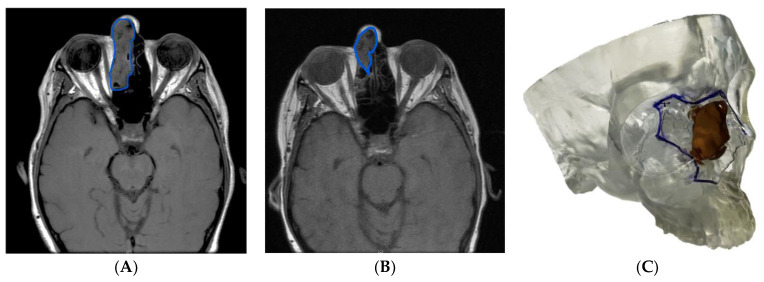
(A) T1-weighted axial MRI pre-induction chemotherapy (blue outline highlighting tumor), (B) T1-weighted axial MRI post-induction chemotherapy, and (C) 3DM showing residual tumor and bony anatomy with planned resection margins.
